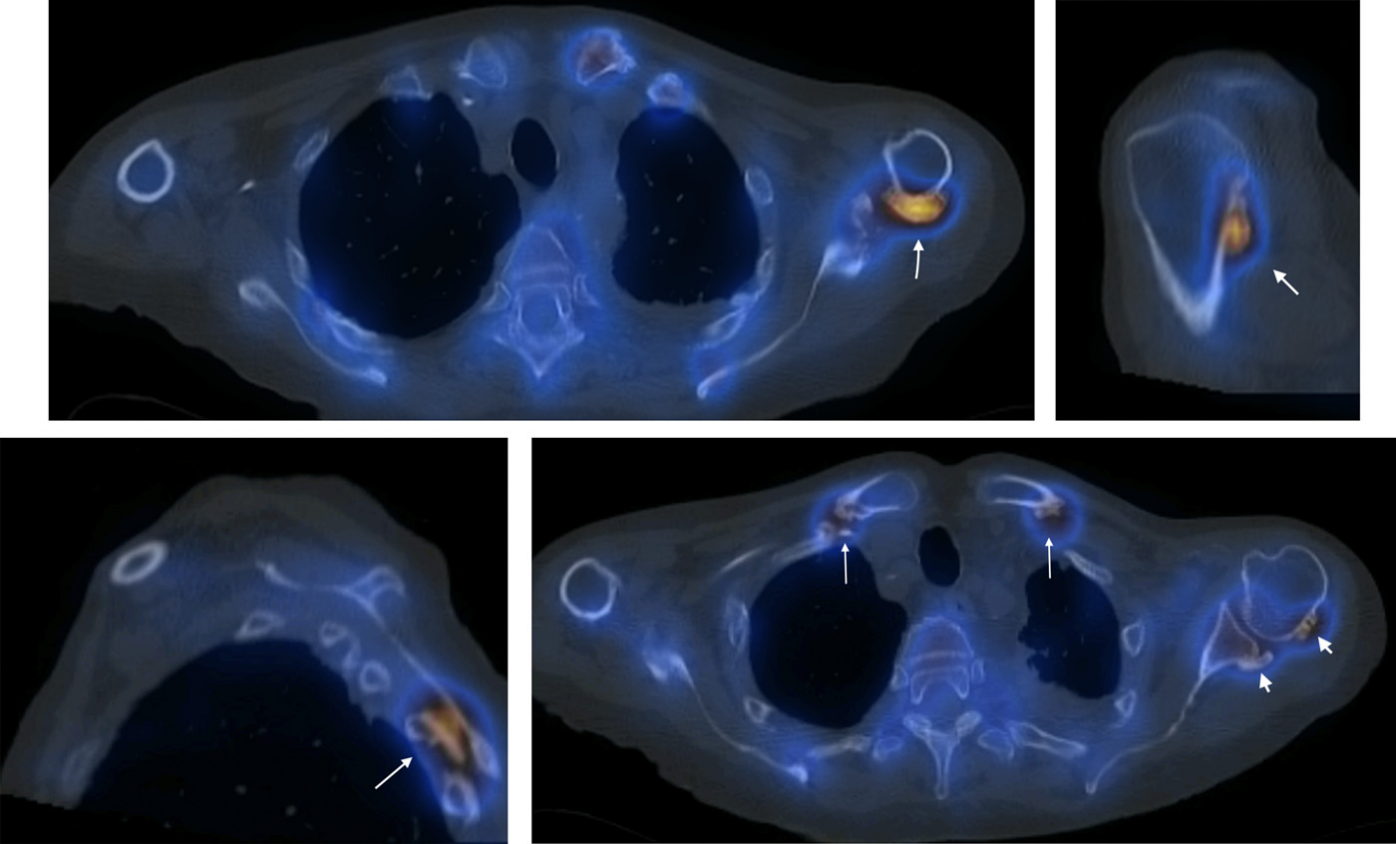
(a). Axial SPECT scan of the thorax demonstrates juxtacortical ossification at the posterior aspect of the proximal left humerus, associated with prominent tracer uptake (white arrow). (b). Parasagittal SPECT image of the left shoulder demonstrates juxtacortical ossification at the posterior aspect of the proximal left humerus, associated with prominent tracer uptake (white arrow). (c). SPECT image at the left parasagittal level shows juxtacortical ossification with tracer uptake in a left posterior rib (white arrow). (d). Axial SPECT image of the upper thorax demonstrates juxtacortical ossification bilaterally at the clavicles (white arrows) and at the left posterior humoral head and left glenoid with low grade increased tracer activity (arrowheads).
